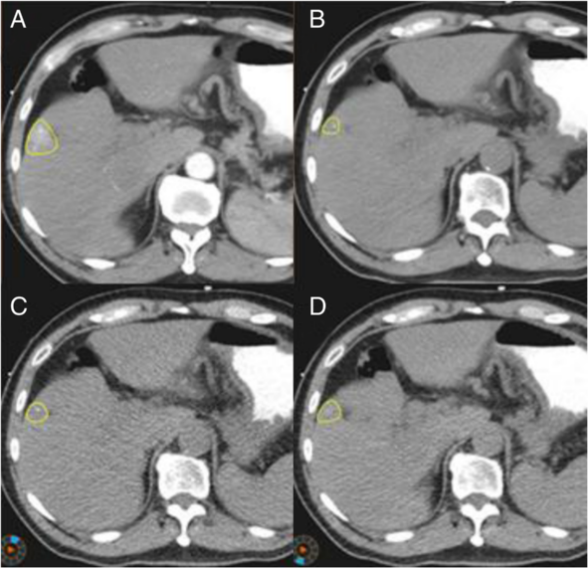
GTV contours obtained from 3D or 4D CT scans before DR (on axial view). A. contrast-enhanced 3D CT image. B. non-contrast-enhanced 3D CT image. C. CT_00_ respiratory phase of 4D CT images. D. CT_50_ respiratory phase of 4D CT images. Yellow contours: GTVs before DR.
